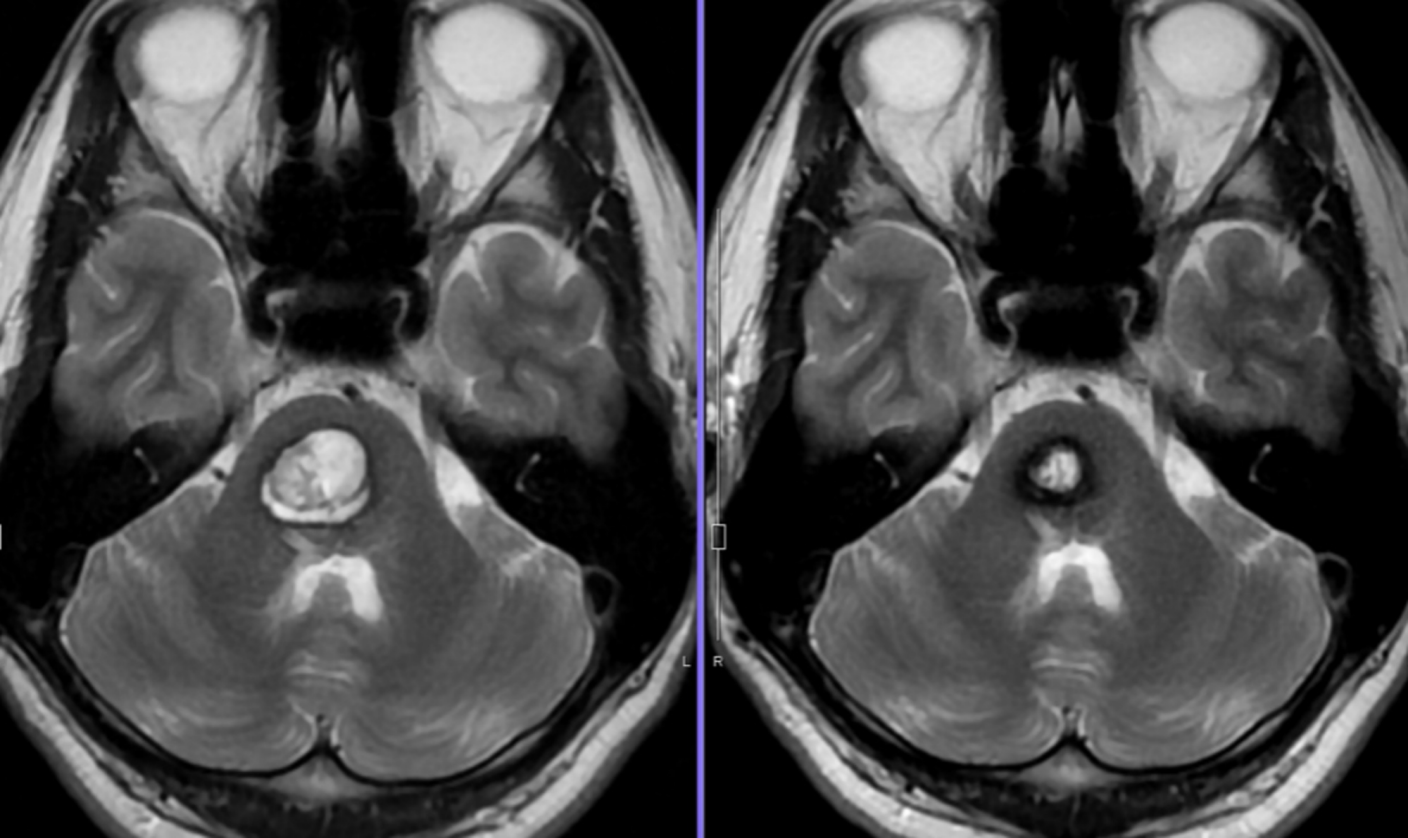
Follow-up MRI findings after radiosurgery A large lesion of cavernous malformation in the center of the brainstem (left) is much smaller in size six months later.
